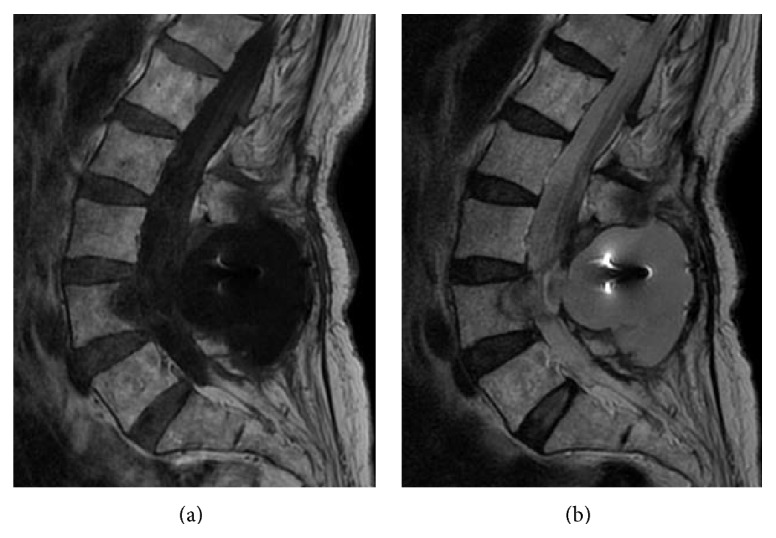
Cerebrospinal fluid accumulation 2 years after surgery. Sagittal images show low intensity on the T1-weighted images (a) and high intensity fluid on the T2-weighted images (b) 2 years after surgery.
